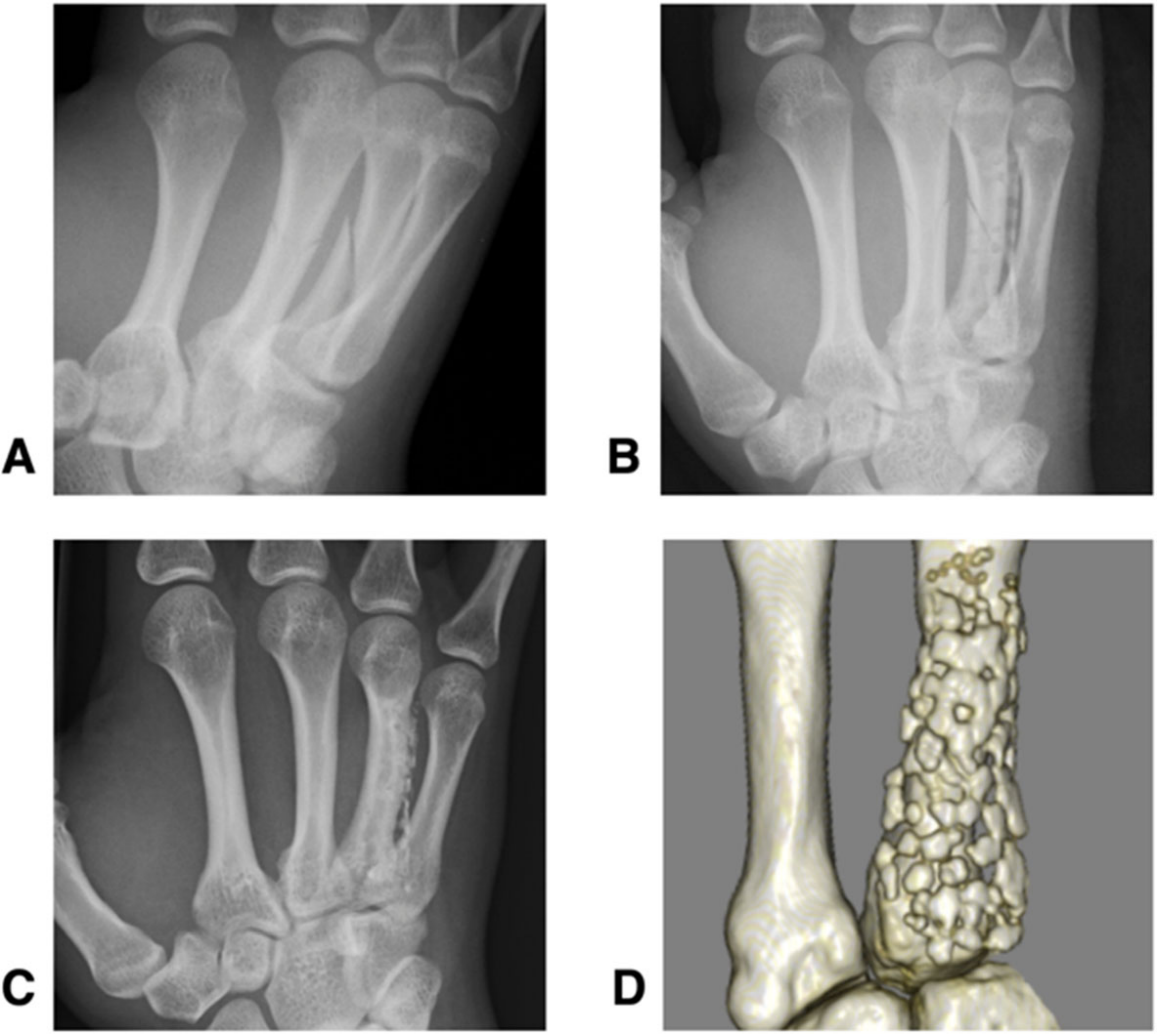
Radiographic images of a 17-year-old male who had an oblique metacarpal fracture of the right ring finger (Case 3). The preoperative radiograph (a), postoperative radiograph (b), final follow-up radiograph (c) (taken 6 years 7 months post-surgery) and a final follow-up 3D-computed tomography image (d) are shown
